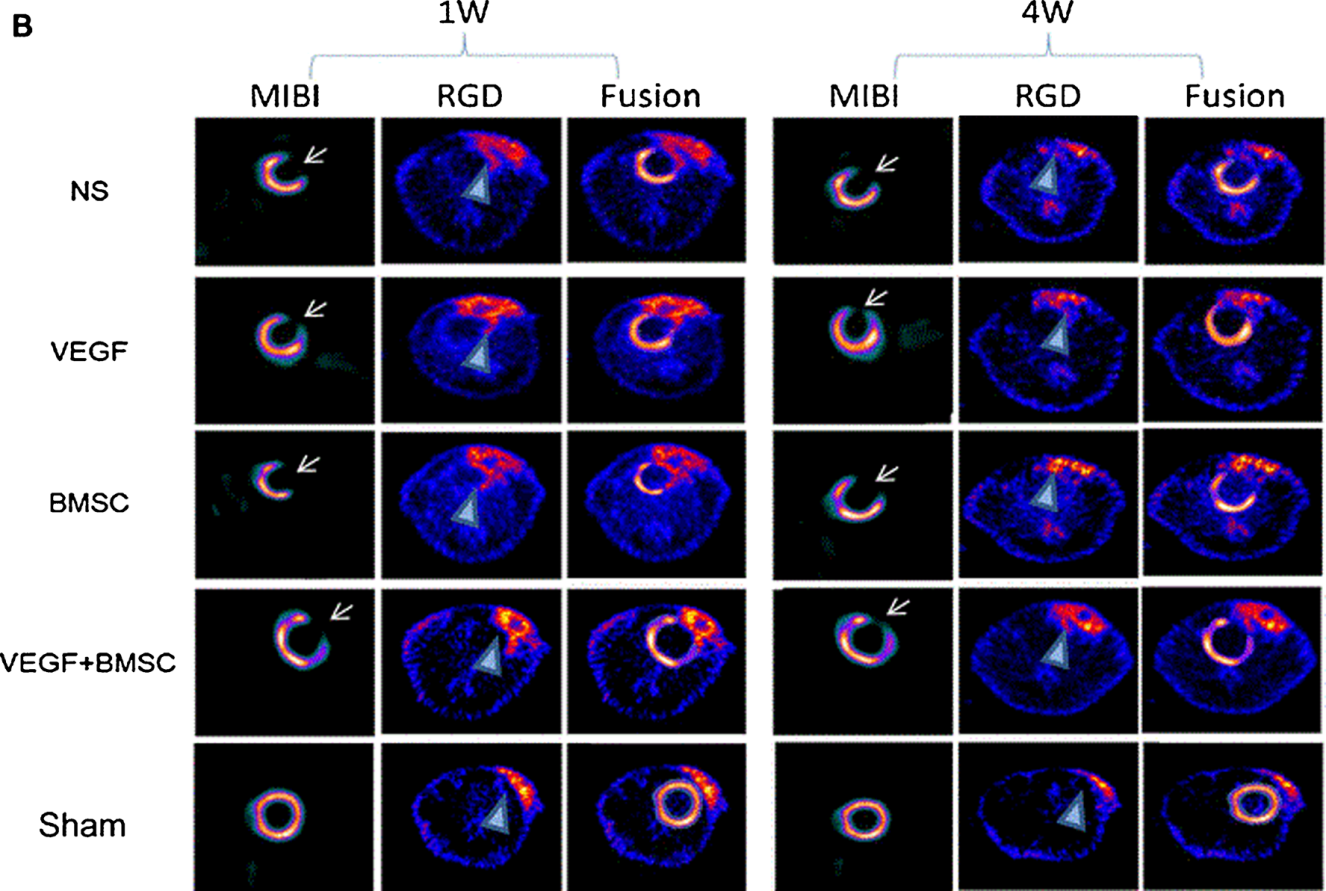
[…] b In vivo PET images of 18F-Alfatide II and representative SPECT myocardial short axis slice images using 99mTc-MIBI at different times after myocardial infarction. Infarcted myocardium showed obvious 99mTc-MIBI uptake defect in the anterior and lateral wall of left ventricle (*arrows*), which matched the focal RGD peptide tracer uptake region (*triangle*). c The infarct area/remote area ratio of 18F-Alfatide II uptake as measured by PET (reprinted from [20•] with permission from Springer)
